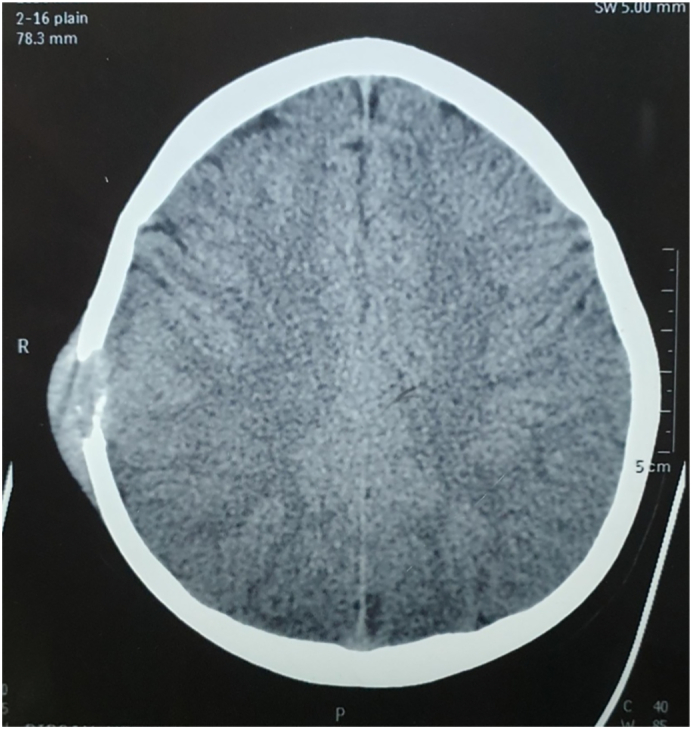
CT scan of the head revealed an approximately 3 cm sized defect involving both inner and outer table in the right temporoparietal calvarial with scalloped margin.
